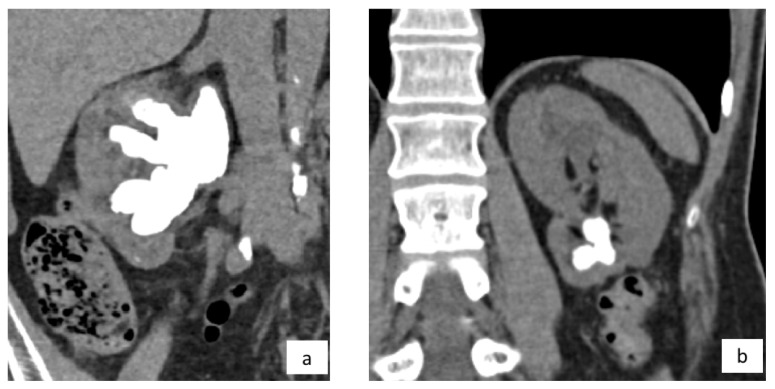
Staghorn calculi on CT scan. (a) Complete staghorn stone occupying the whole pyelocaliceal cavities; (b) Partial lower pole staghorn calculus.
